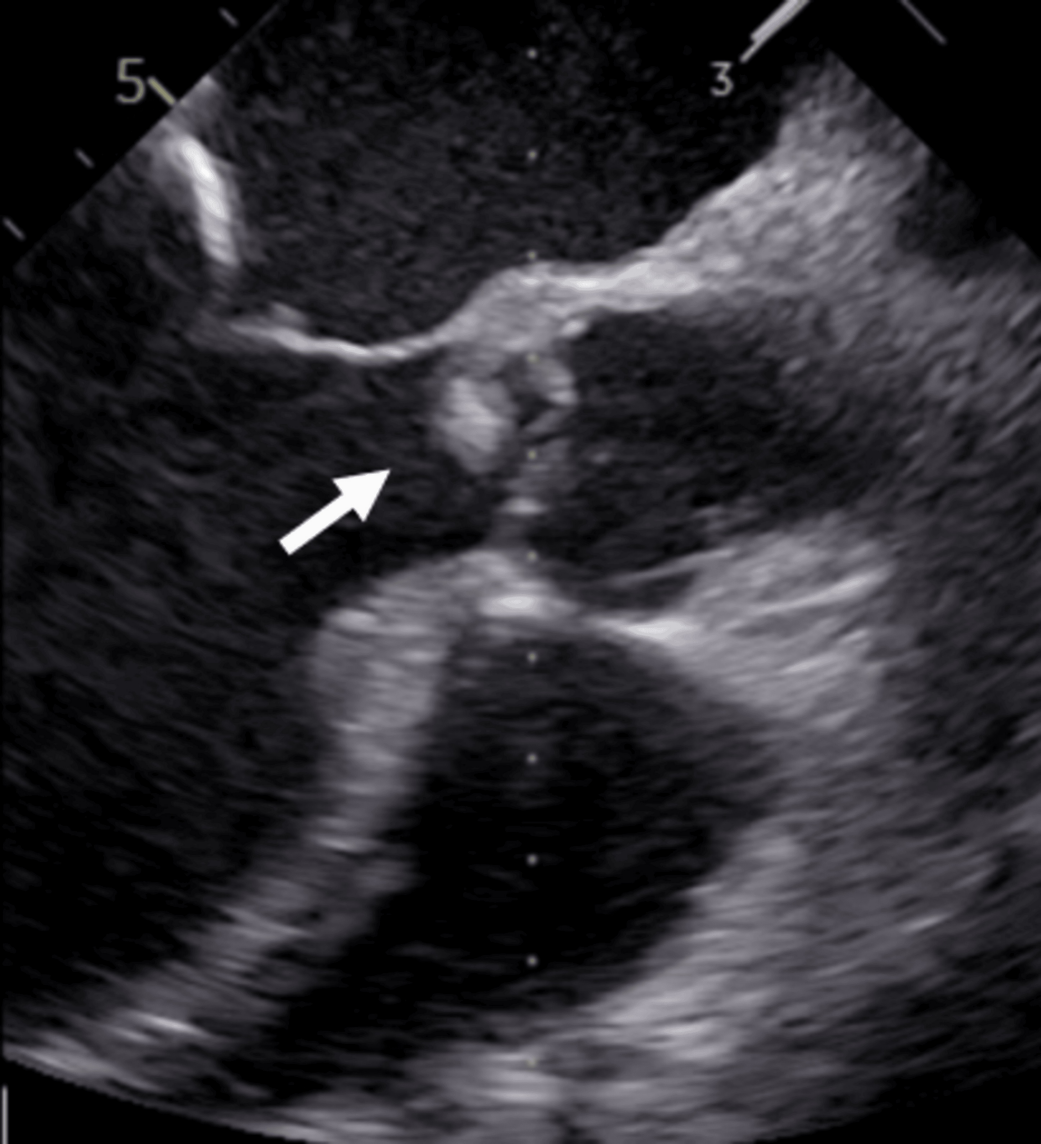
Aortic valve vegetation on TEE (indicated by arrow). TEE, transesophageal echocardiography. Transesophageal echocardiogram of a 64-year-old man showing vegetation on the aortic valve.
